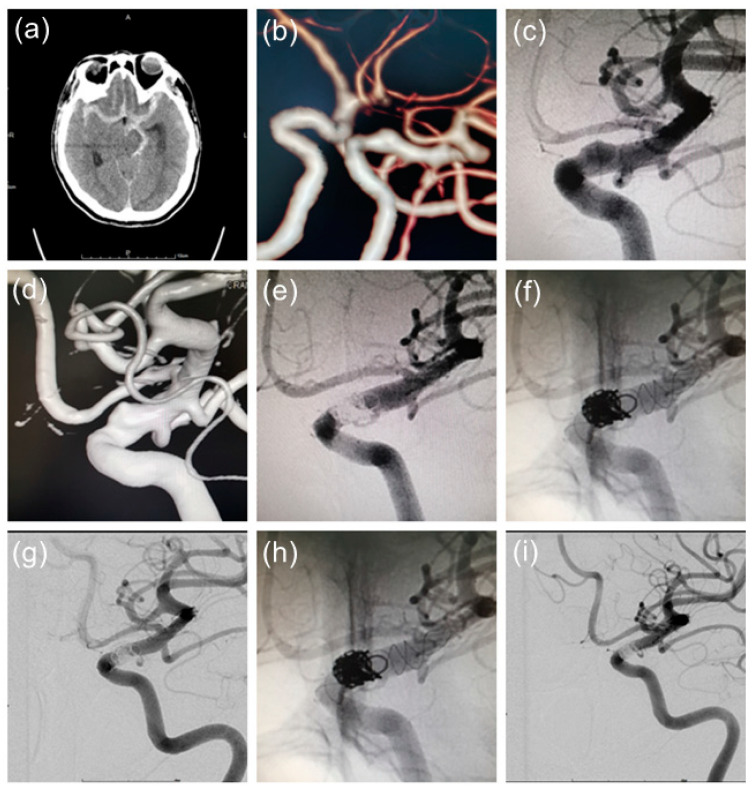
Thirty-six-year-old male presented with a severe, acute headache, accompanied by a brief period of unconsciousness, and an axial CT head showed a subarachnoid hemorrhage (a). A pretreatment CT angiogram exhibited an aneurysm in the clinoid segment of the left intracranial artery (b). DSA examination and 3D reconstruction cleared the ruptured blood blister-like aneurysm (treatment angle) (c,d). During the process, the patient underwent FDD-assisted coiling via a semi-deploying method (Tubridge 3.5 × 25 mm). After treatment, the aneurysm was completely occluded (e,f). Follow-up angiograms at 3 months (g,h) and 6 months post-procedure showed complete resolution of the aneurysm with remodeling of the parent vessel (i).
